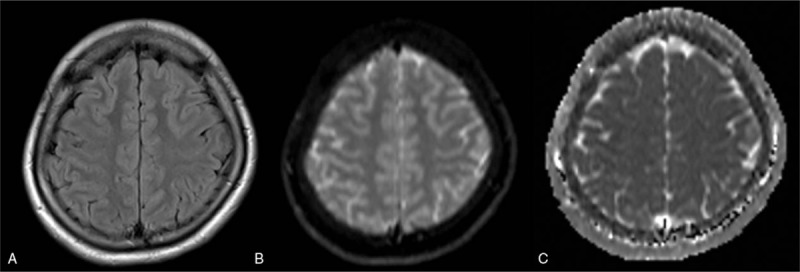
One month after admission. MRI axial T2-weighted FLAIR (A), DWI (B), and ADC mapping (C) showed complete remission of the foci. ADC = apparent diffusion coefficient, DWI = diffusion-weighted magnetic resonance imaging, FLAIR = fluid-attenuated inversion recovery, MRI = magnetic resonance imaging.
